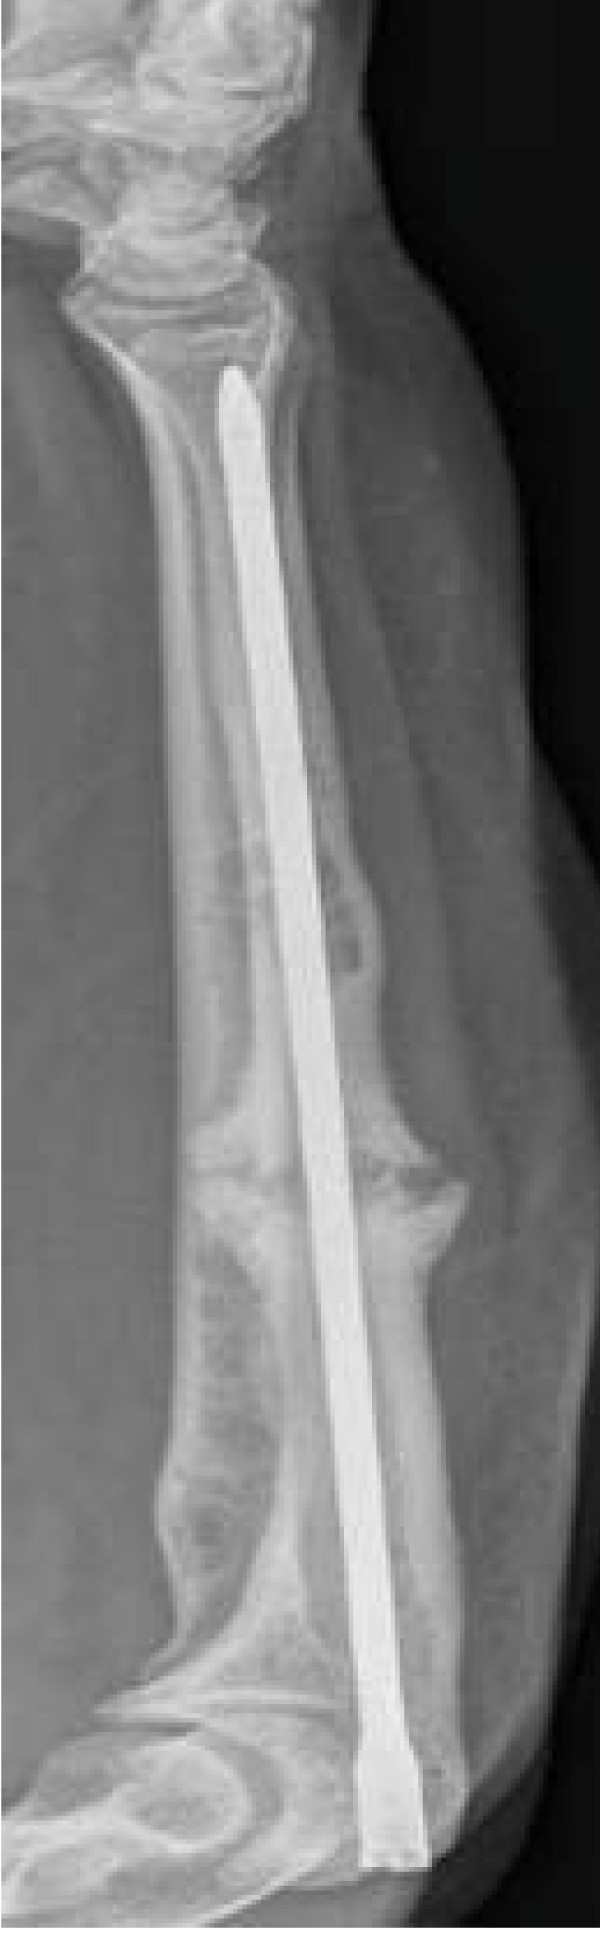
Anteroposterior X-Ray view of intramedullary fixation of pathological fracture of the right ulna in the immediate postoperative period.
